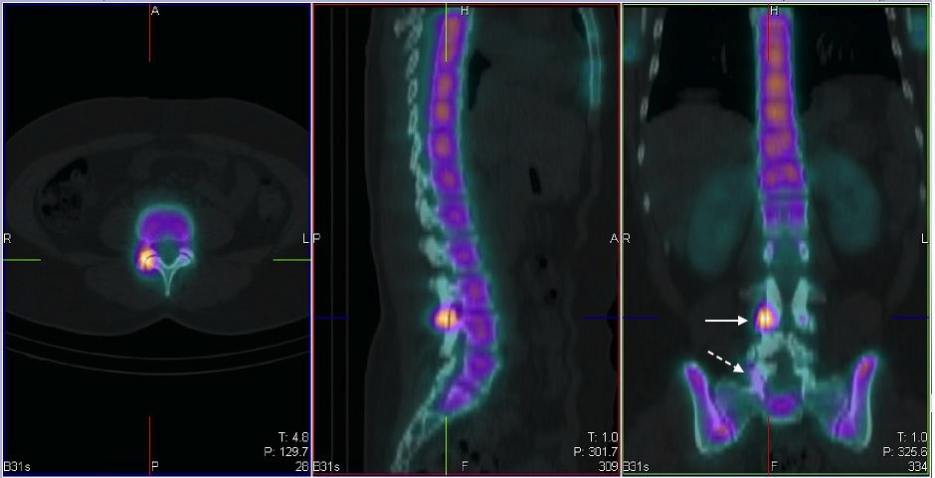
SPECT/CT images (axial, sagittal, coronal) localizing intense, focal tracer uptake to the right L3/L4 facet joint (continuous arrow). Note is made of mild tracer uptake in the right L5/S1 facet joint (dashed arrow).
